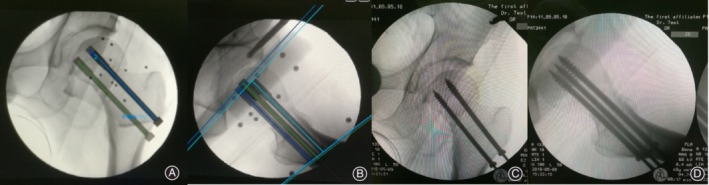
The surgeon could place the screw in the desired position through the workstation of the system (A, B). The robot arm would then provide a guide channel to help the surgeon achieve screw placement in a precise position. From the intraoperative anteroposterior (C) and lateral (D) radiographs, we can see that the screws were placed in the surgeon's planned position.
